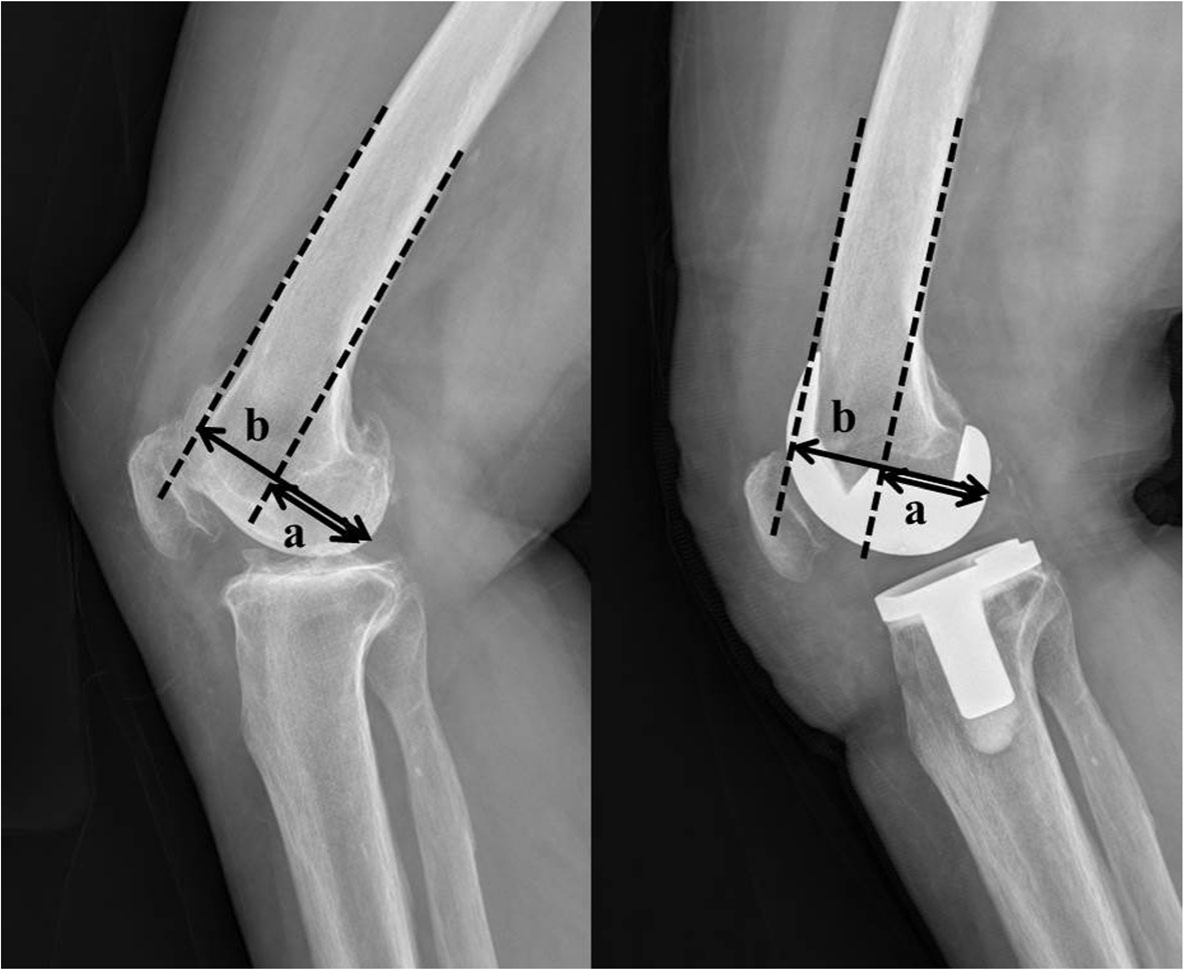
Measurement of the posterior condylar offset (PCO, a) and posterior condylar offset ratio (PCOR, a/b) based on true lateral preoperative and postoperative knee radiographs. a distance in millimeters from the tangent of the femoral diaphysis posterior cortex to the posterior condylar margin; b distance in millimeters from the posterior condylar border to the tangent of the femoral diaphysis anterior cortex
